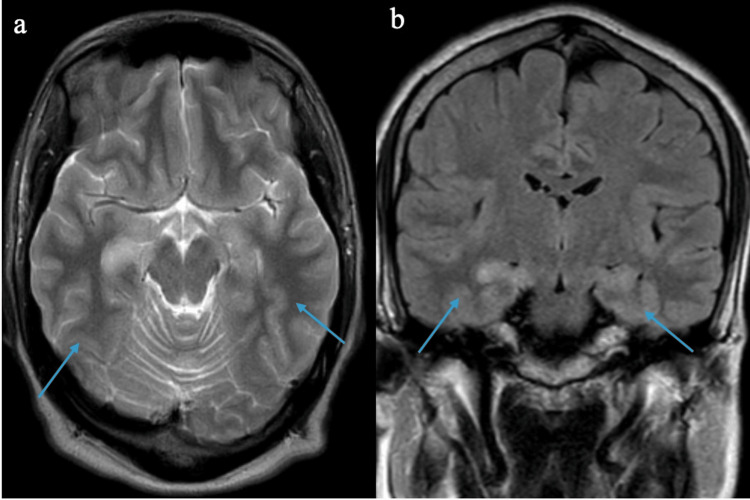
MRI showing bilateral medial temporal lobe abnormalities with hyperintensities
